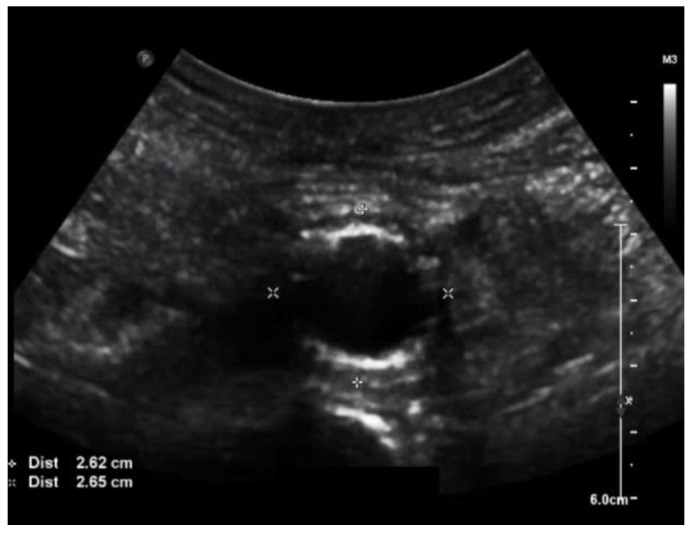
Duplex ultrasound at 6 months after endovascular repair of the mycotic aneurysm, demonstrating a stable sac that had decreased to a size of 2.65 cm.
